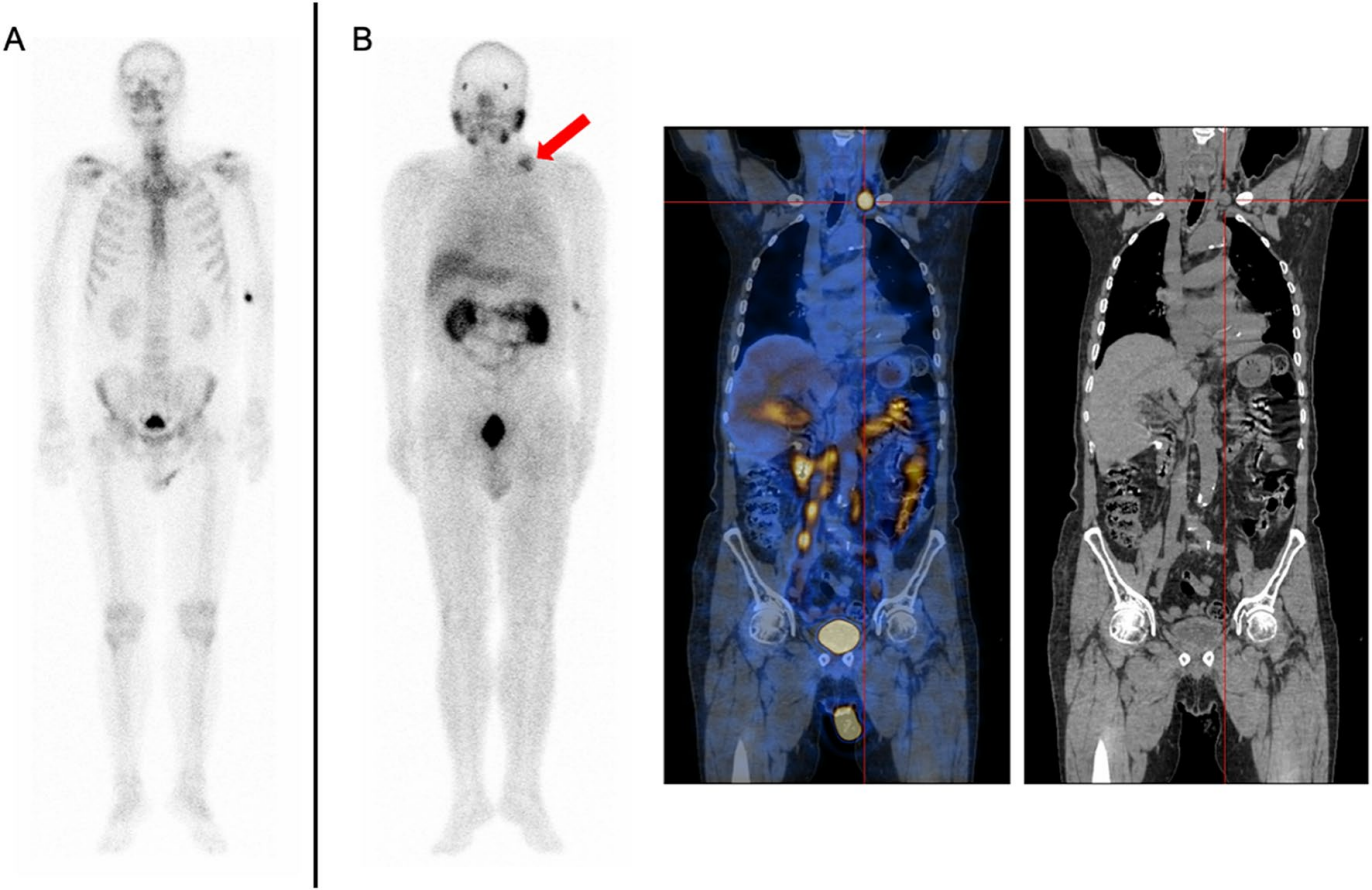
A 67-year-old patient with a restaged PCa. The Gleason score was 10, and the PSA level was 0.11 ng/mL; (A): Whole-body planar ^99m^Tc-MDP. (B): Whole-body planar ^99m^Tc-PSMA and coronal SPECT/CT. ^99m^Tc-PSMA SPECT/CT revealed lymph node metastases in the left supraclavicular fossa (B, red arrow and cross).
